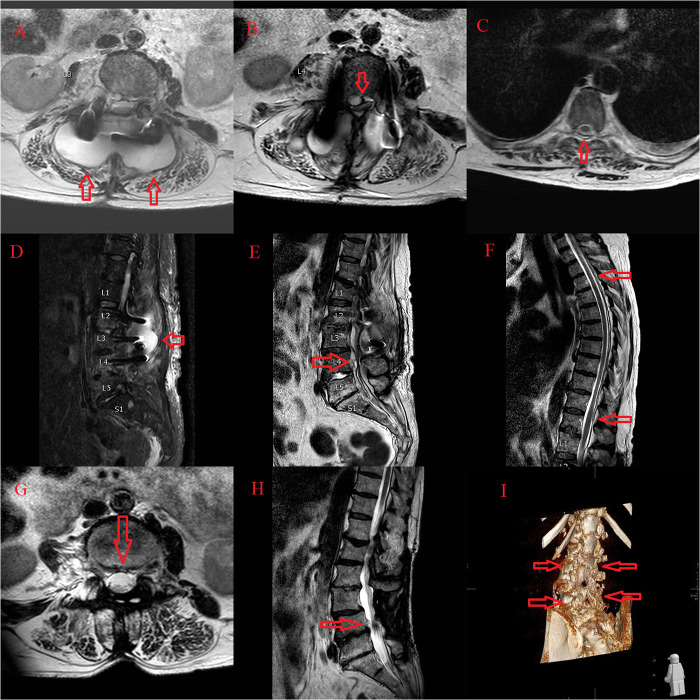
Emergent MRI demonstrated pyogenic spondylodiscitis with paraspinal and epidural purulent collection at the level of spondylodesis L2 to L4 (A,D) with total spinal stenosis at the L4 level (B,E). Concomitant purulent collections, without neurologic compromise, from the Th4 to Th12 level (C,F). The follow-up imaging revealed residual fluid collections in the paraspinal soft tissue and right psoas muscle, with regression of spondylodiscitis, resolution of total spinal stenosis at the L4 level (G,H), and evidence of bone fusion with postoperative deformity at the level of surgery (I).
